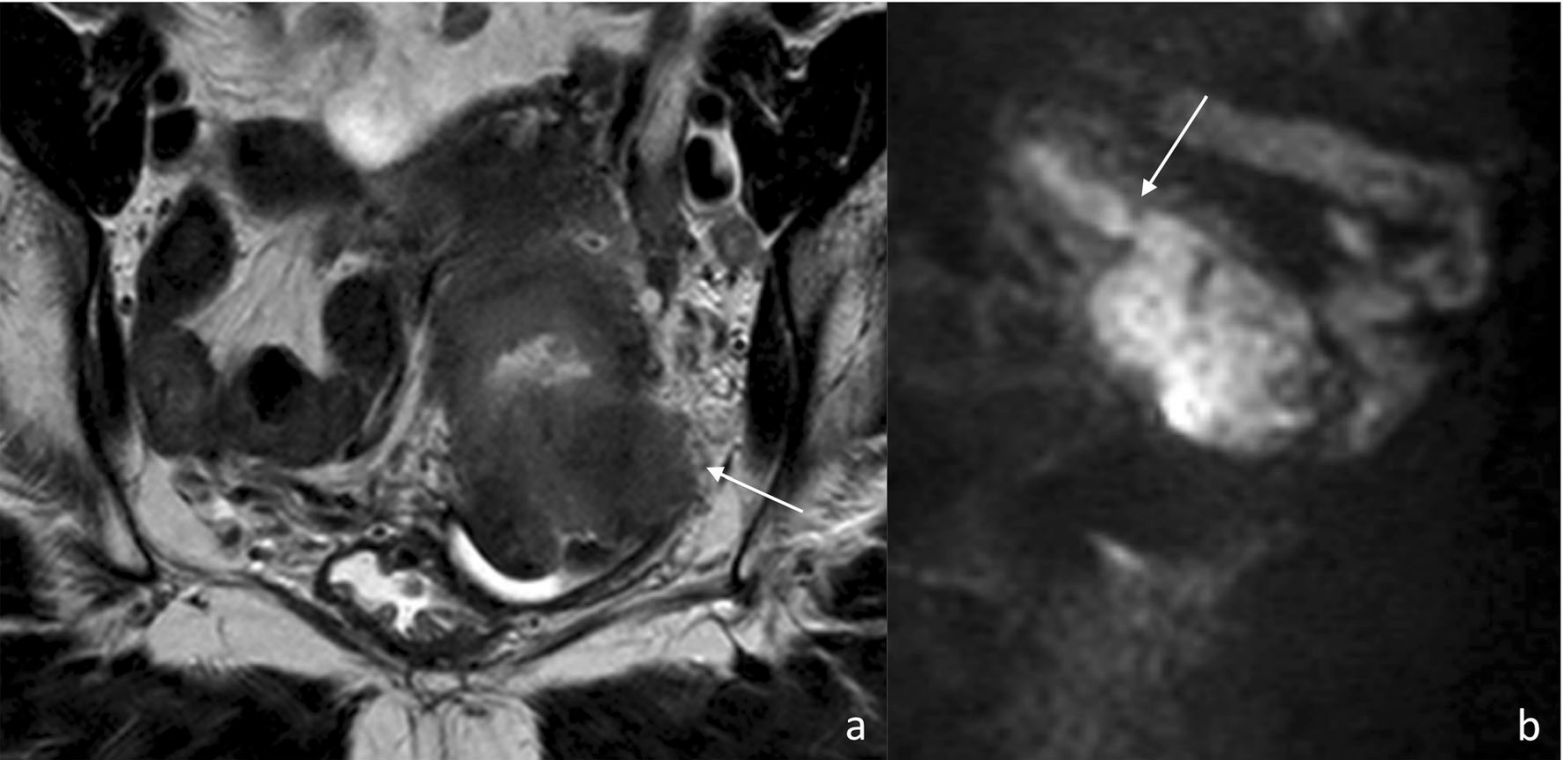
Seventy-two-year-old female patient with stage IIb cervical cancer, as seen on oblique axial high resolution T2-weighted (a) and sagittal diffusion-weighted (b) images. A bulky cervical mass invades the left subperitoneal mesometrium to its lateral limit, clearly not extending beyond it (*arrow*); therefore, respecting lower Mullerian compartment boundaries (a). In the sagittal plane (b), the mass is seen to invade the uterine corpus (*arrow*), contained in the closely related middle Mullerian compartment
